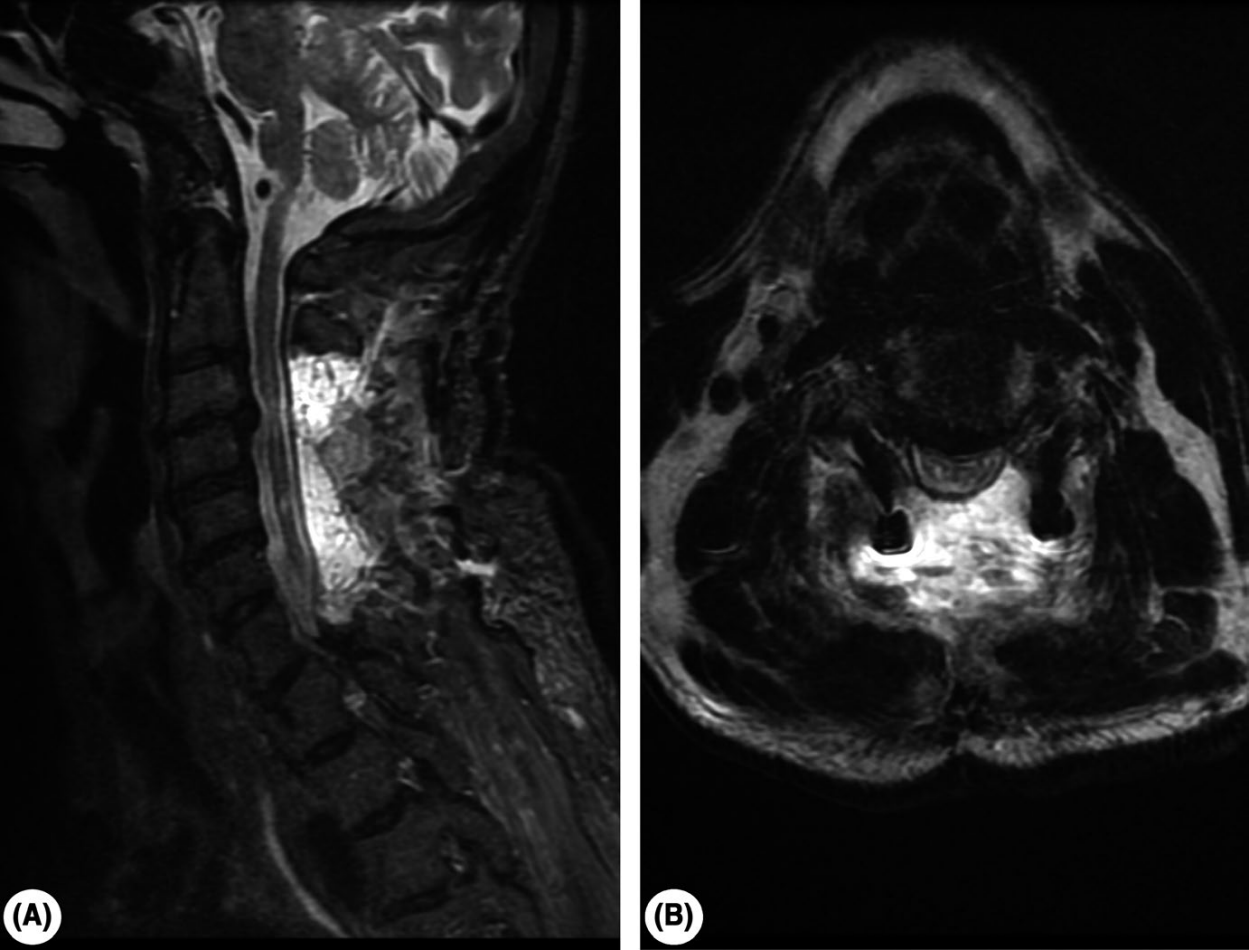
Postoperative cervical nonenhanced MRI aspect. A, Sagittal STIR sequence showing decompression of the spinal cord through laminectomy, with posterior postoperative edema. B, Axial T2‐weighted frFSE sequence, indicating reversal of the conflict on spinal cord and spinal nerve roots, with postoperative edema and artifacts from osteosynthesis materials
